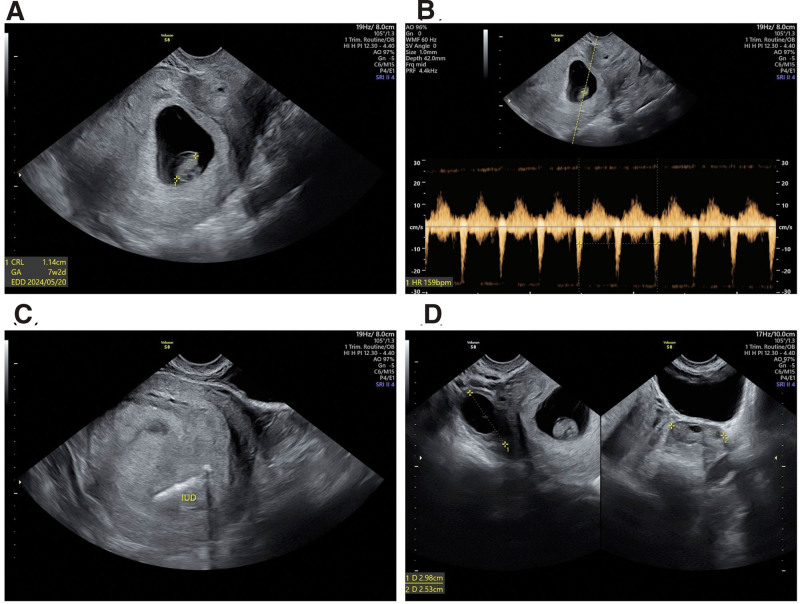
Transvaginal ultrasound of the pregnancy with an IUD in place. (A) Crown-rump length of the fetus. (B) Fetal heartbeat of 159 bpm. (C) The IUD is located in the placental region. (D) Bilateral adnexa are in normal appearance.
